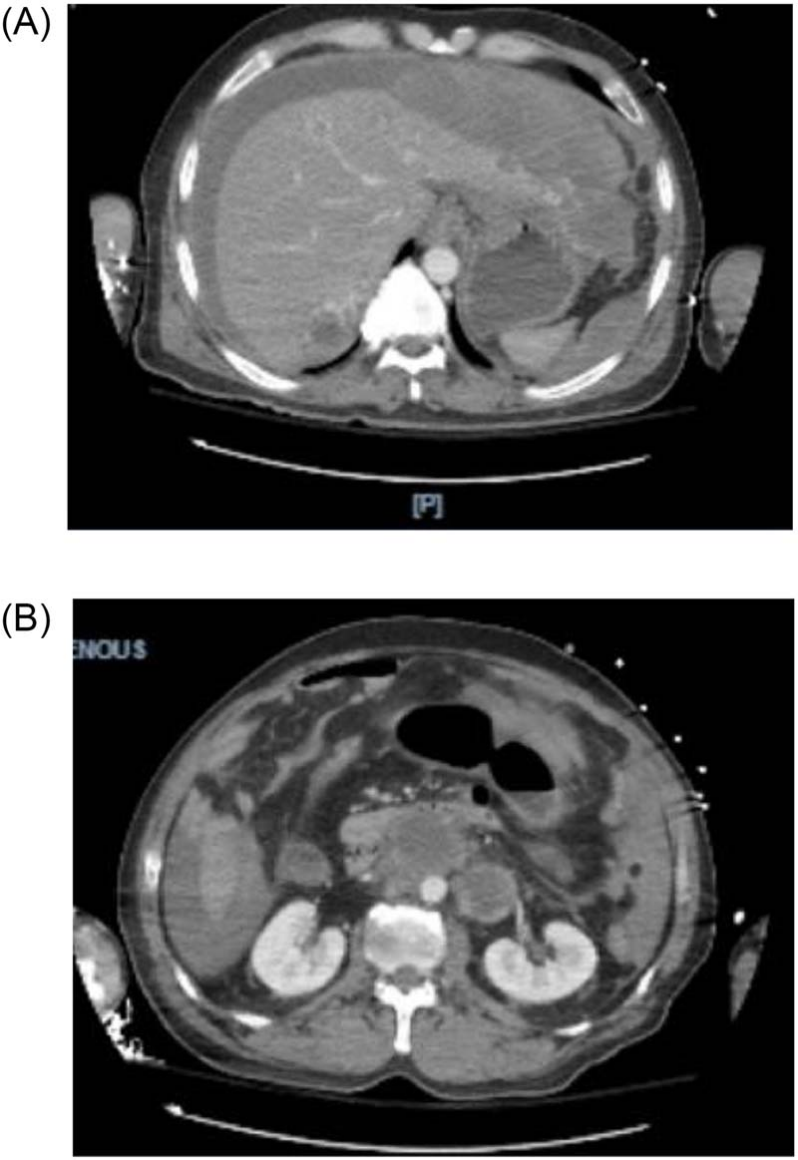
(A, B) CTA shows hemoperitoneum anterior to the left lobe of the liver.
